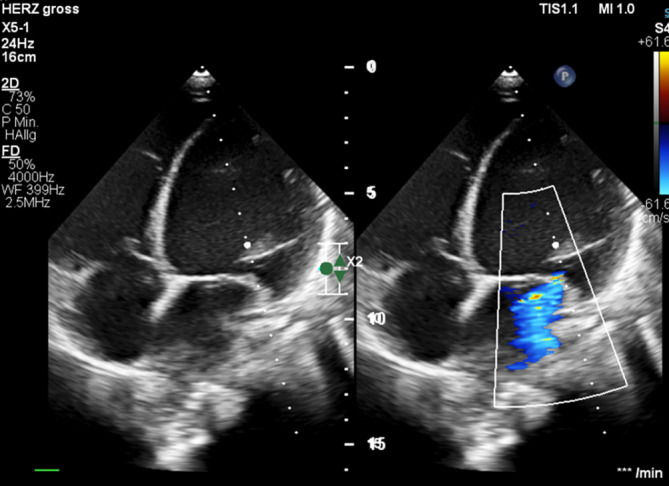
Echocardiography on admission. Note left ventricular dilatation and mitral valve insufficiency
